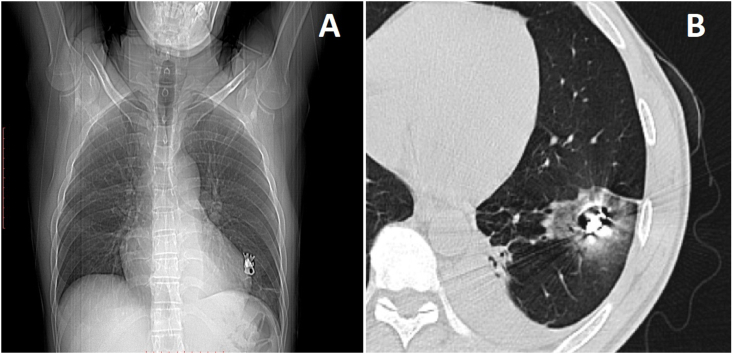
(A) Chest radiograph showing a 2-cm-long chainsaw tooth in the left hemithorax. (B) Computed tomography image showing a left-sided pleural effusion and a pulmonary injury of the left lower lobe.
